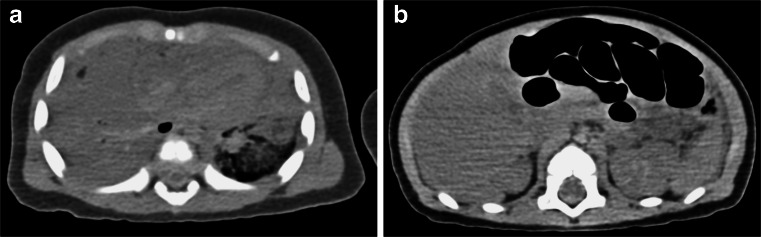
Unenhanced postmortem CT in a 14 day old female infant who died unexpectedly, the cause of death was unascertained. Unenhanced postmortem axial CT of the chest (a) and abdomen (b) in a neonate demonstrate poor signal contrast, with limited differentiation between heart and non-aerated lungs, and abdominal organs
